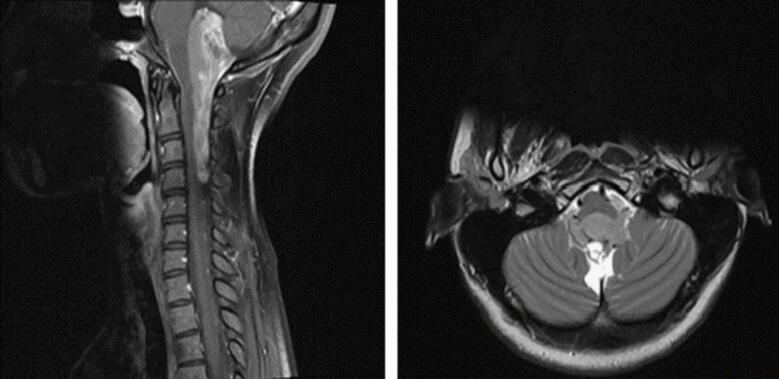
MRI brain and cervical spine demonstrating a posterior fossa tumor extending downward past the foramen magnum to the C4 vertebral body, on T1 weighted post gadolinium sagittal view and T2 weighted axial view, respectively.
